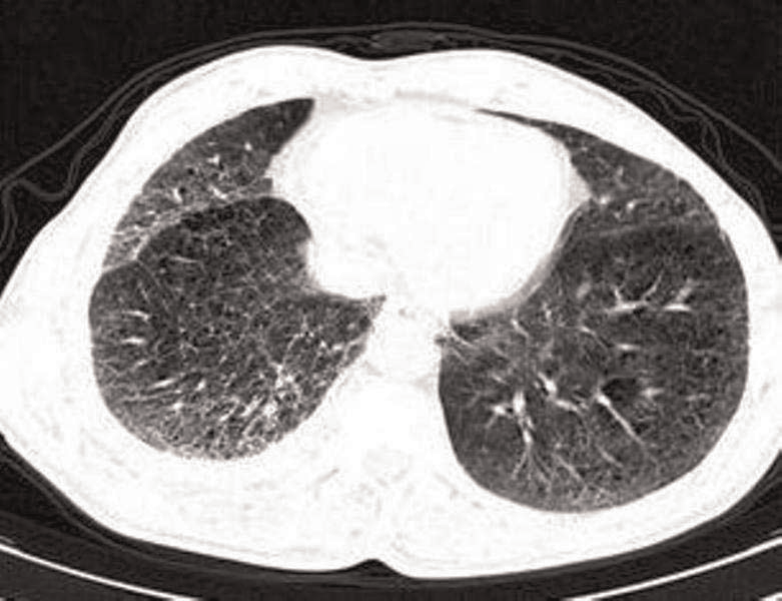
HR-CT showing diffuse ground glass opacities predominantly involving the lung basis. This aspect being consistent with the clinical finding of fine inspiratory basal crackles at auscultation.
